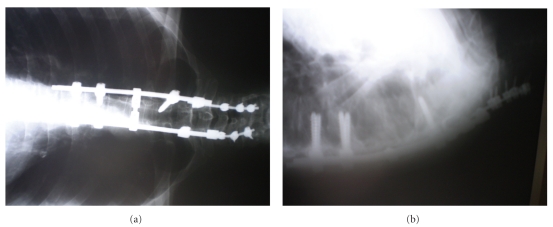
The postoperative plain X-ray.
